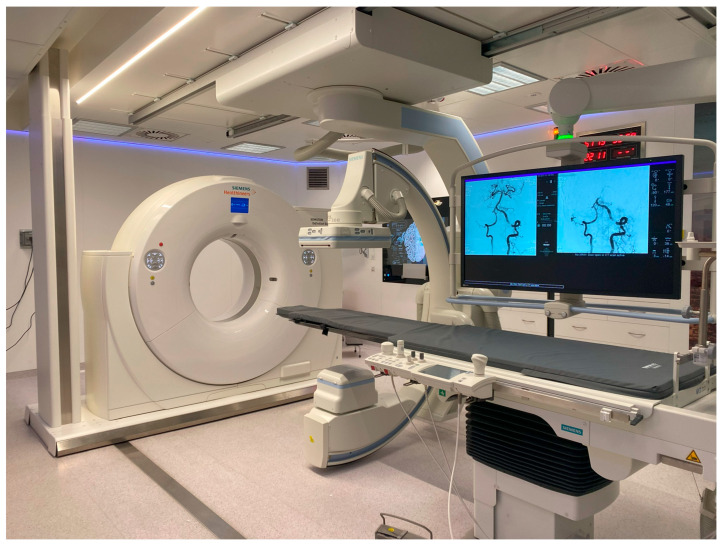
Hybrid CT angio suite (Nexaris Angio-CT, Siemens).
